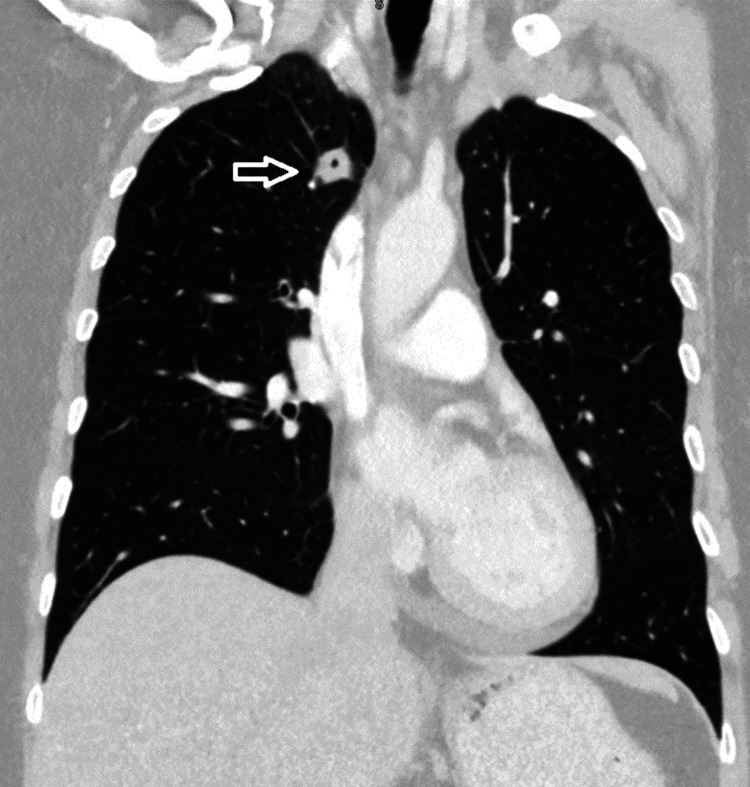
Coronal CT imaging of the lung showing a cavitating lesion in the right upper lobe (white arrow).
